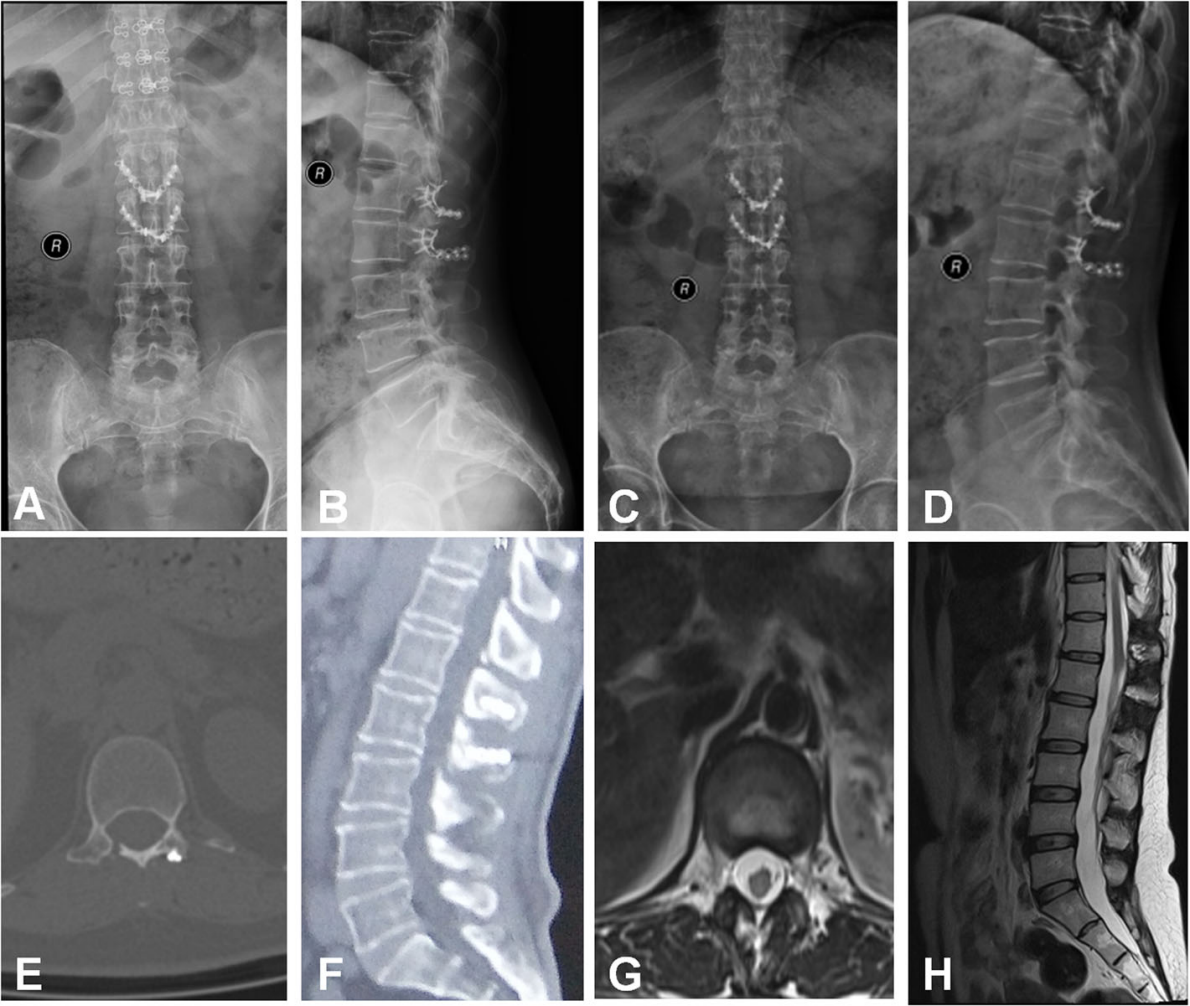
Patient with intraspinal tumour treated by laminoplasty with reconstruction plate fixation. a and b Six months following the surgery, X-ray examination indicated no fixation transposition or fracture, lumbar instability or kyphosis. c and d Twelve months following the surgery, X-ray examination indicated no fixation transposition or fracture, lumbar instability or kyphosis. e and f Six months following the surgery, CT scans indicated favourable osseous union of the replantation lamina. g and h Six months following the surgery, MRI indicated no tumour recurrence, and no intraspinal restenosis or scar adhesions
